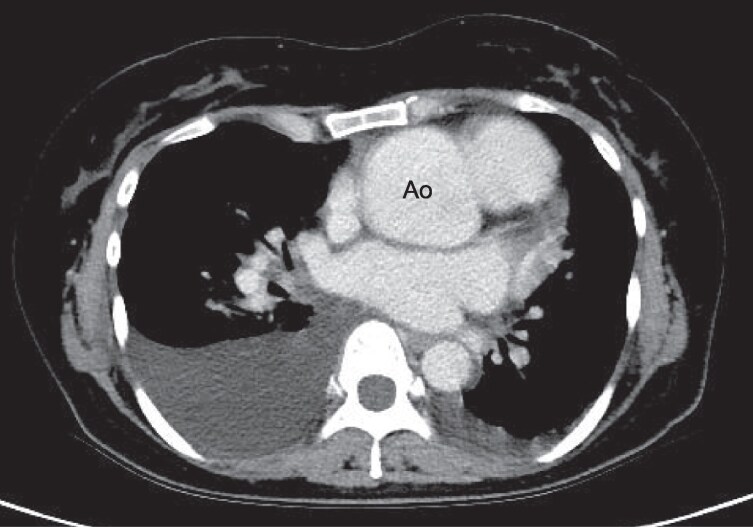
Contrast-enhanced chest computed tomography. Computed tomography showed bilateral pleural effusion and marked dilation of the aortic root (51 mm), but there was no evidence of aortic dissection.
